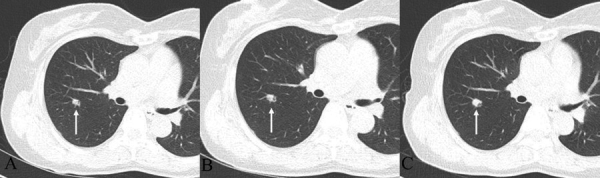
Chest CT with follow up. (A) 9-mm lobulated nodule with an internal air-bronchogram in the right upper lobe. (B) After 1 year, the nodule shows a minimal increase in size, 9 to 10 mm. (C) After 2 years later from the initial chest CT, the nodule changes from 10 to 13 mm in size, and the somewhat dilated internal bronchus are detected.
